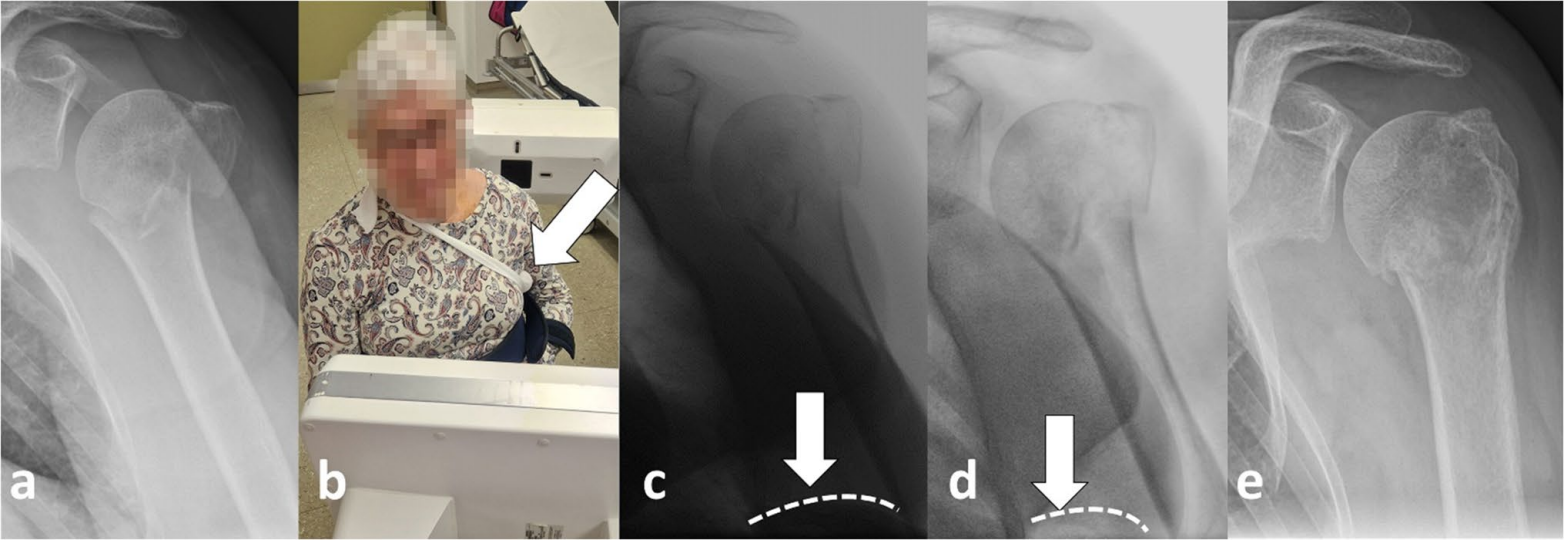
AP radiographs (a + e) and fluoroscopic images (c + d) of a 78-year-old patient with a valgus impacted PHF with medial shaft translation before (a), and immediately after (b + c) application of an arm sling with an axillary roll (white arrow and white dashed line). Fluoroscopic control after 3 weeks (d), and x-ray control after 9 weeks (e) showed a reduced medial hinge by about 5 mm and a reduced head-shaft-angle (HSA) by approximately 15° towads a nearly anatomic HSA
